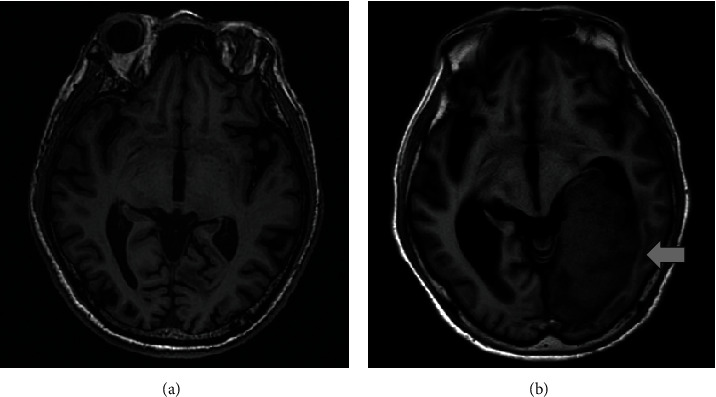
Brain MRI (a) 10 months after and (b) 2 years after mania onset, with a brain mass at 2 years (arrow).
